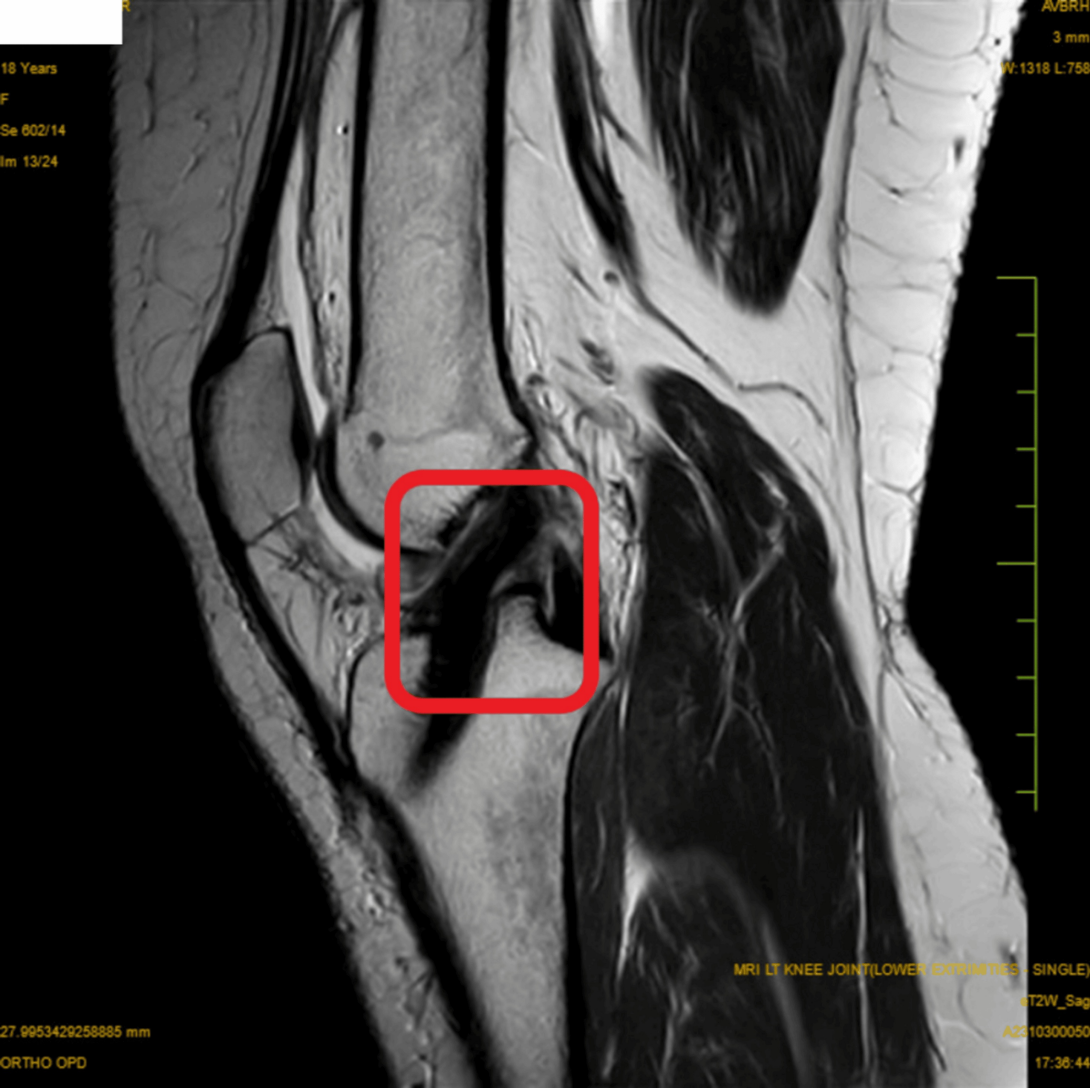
MRI showing the partial anterior cruciate ligament graft tear.
